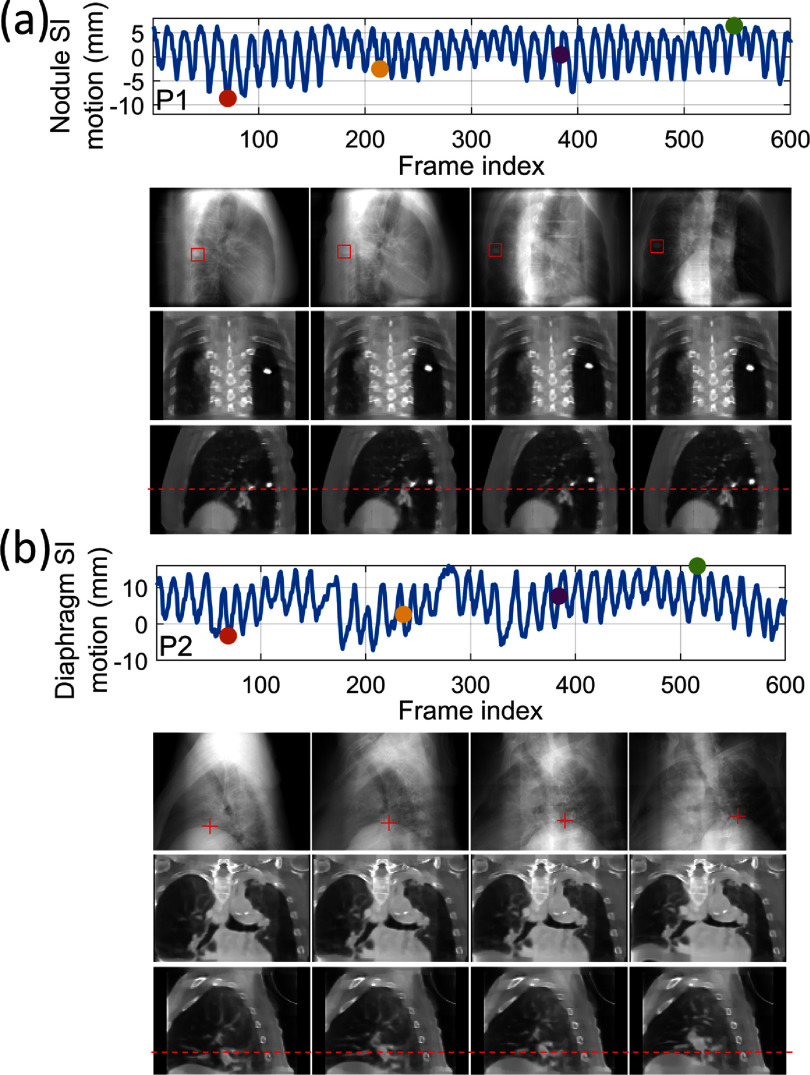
Real-time CBCTs and motion trajectories estimated by DREME for (a) P1 and (b) P2 of the patient study. The first row shows the SI motion trajectory of the tracking landmark (P1: lung nodule; P2: diaphragm apex), with the dots indicating the acquisition time points selected for plotting. The second row presents the onboard x-ray projections at the selected time points, and the ‘□’ and ‘+’ symbols indicate the 2D-projected landmark positions solved by DREME. In the following rows, real-time CBCTs at the selected time points are presented in the coronal and sagittal views.
